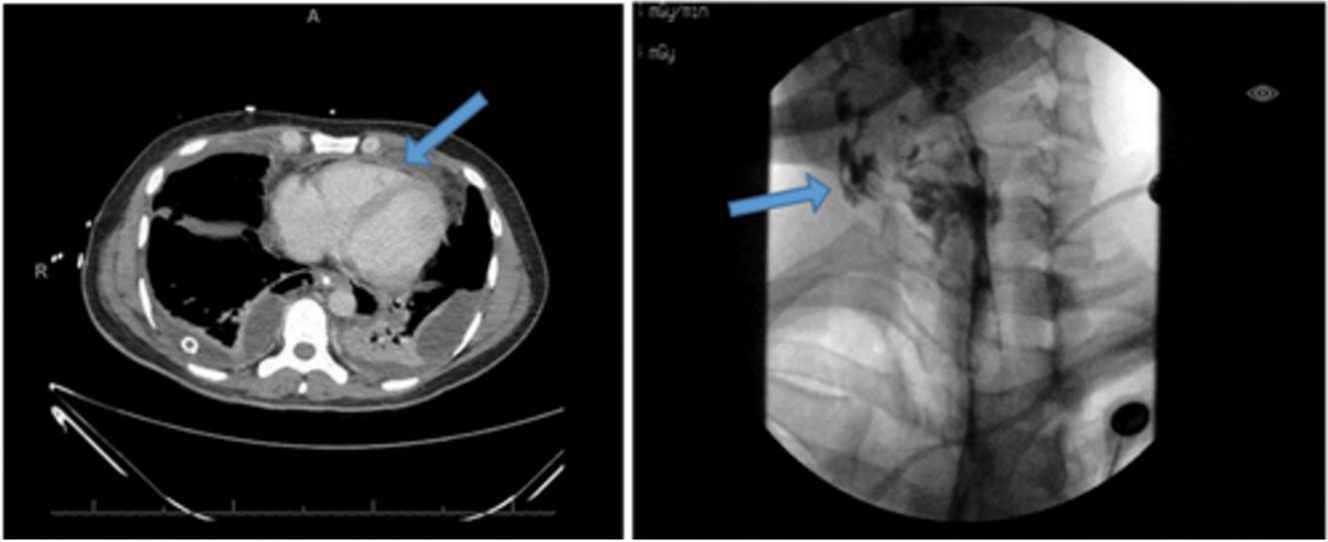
(*A*) CT chest revealing for bilateral effusion of the posterior lung fields and trace effusion of the anterior mediastinum (arrow) surrounding the pericardium, (*B*) still imaging from oesophagram prior to development of tachyarrhythmia with extravasated fluid visualized within the mediastinum (arrow).
